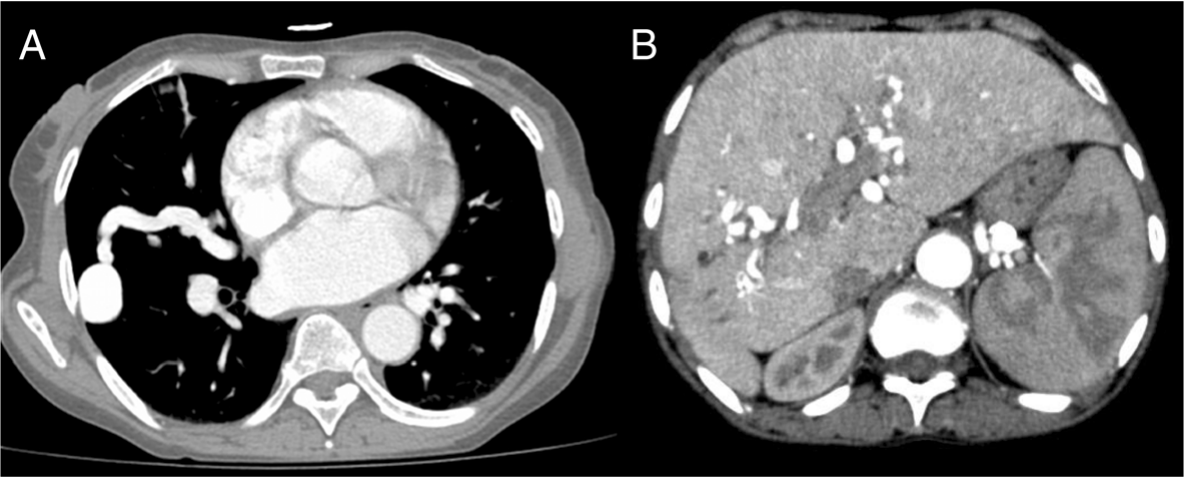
Computed tomography of the chest demonstrating a large pulmonary arteriovenous malformation (a) and multiple hepatic arteriovenous malformations (b). Contrast-enhanced computed tomographic scans show (a) a dilated vessel in the right midperipheral lung region creating a pool of contrast, as well as a large draining vein into the right pulmonary vein suggestive of an arteriovenous malformation, and also show (b) multiple intrahepatic tortuous vessels enhancing on arterial phase suggestive of intrahepatic arteriovenous malformations and peripheral smaller ones with early venous phase displaying early filling of the hepatic veins. The splenic hilum also shows tortuous vessels suggestive of splenic hilar arteriovenous malformations
